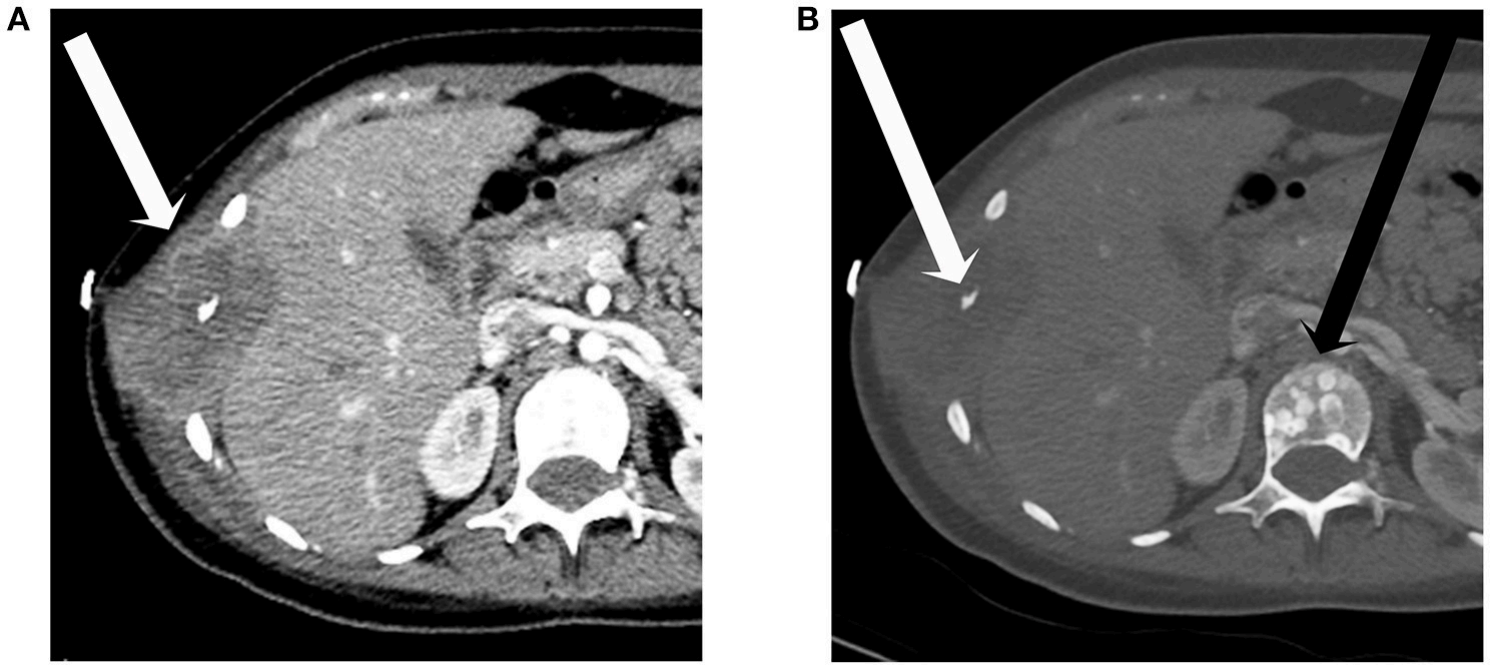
A 17-year-old female with H3F3A G34R mutated infiltrative glioma. An axial image from a CT of the chest with contrast in soft tissue window (A) reveals a destructive soft tissue lesion involving a lower right rib (arrow). The image in bone window (B) better demonstrates the rib destruction (white arrow) and also shows predominantly blastic lesions within the vertebra (black arrow).
